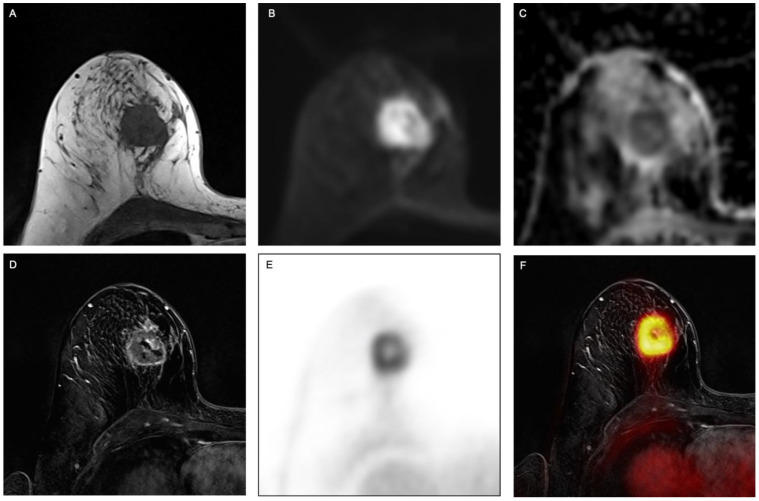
Example of a 63-year-old woman with invasive breast cancer in the right breast, clearly visible on (A) fat-saturated T2-weighted turbo spin-echo (TSE) sequence, (B) transversal diffusion-weighted echo-planar imaging (EPI) sequence with (C) apparent diffusion coefficient (ADC) as well as on (D) contrast-enhanced T1w images, (E) PET and (F) fused PET/MR images.
